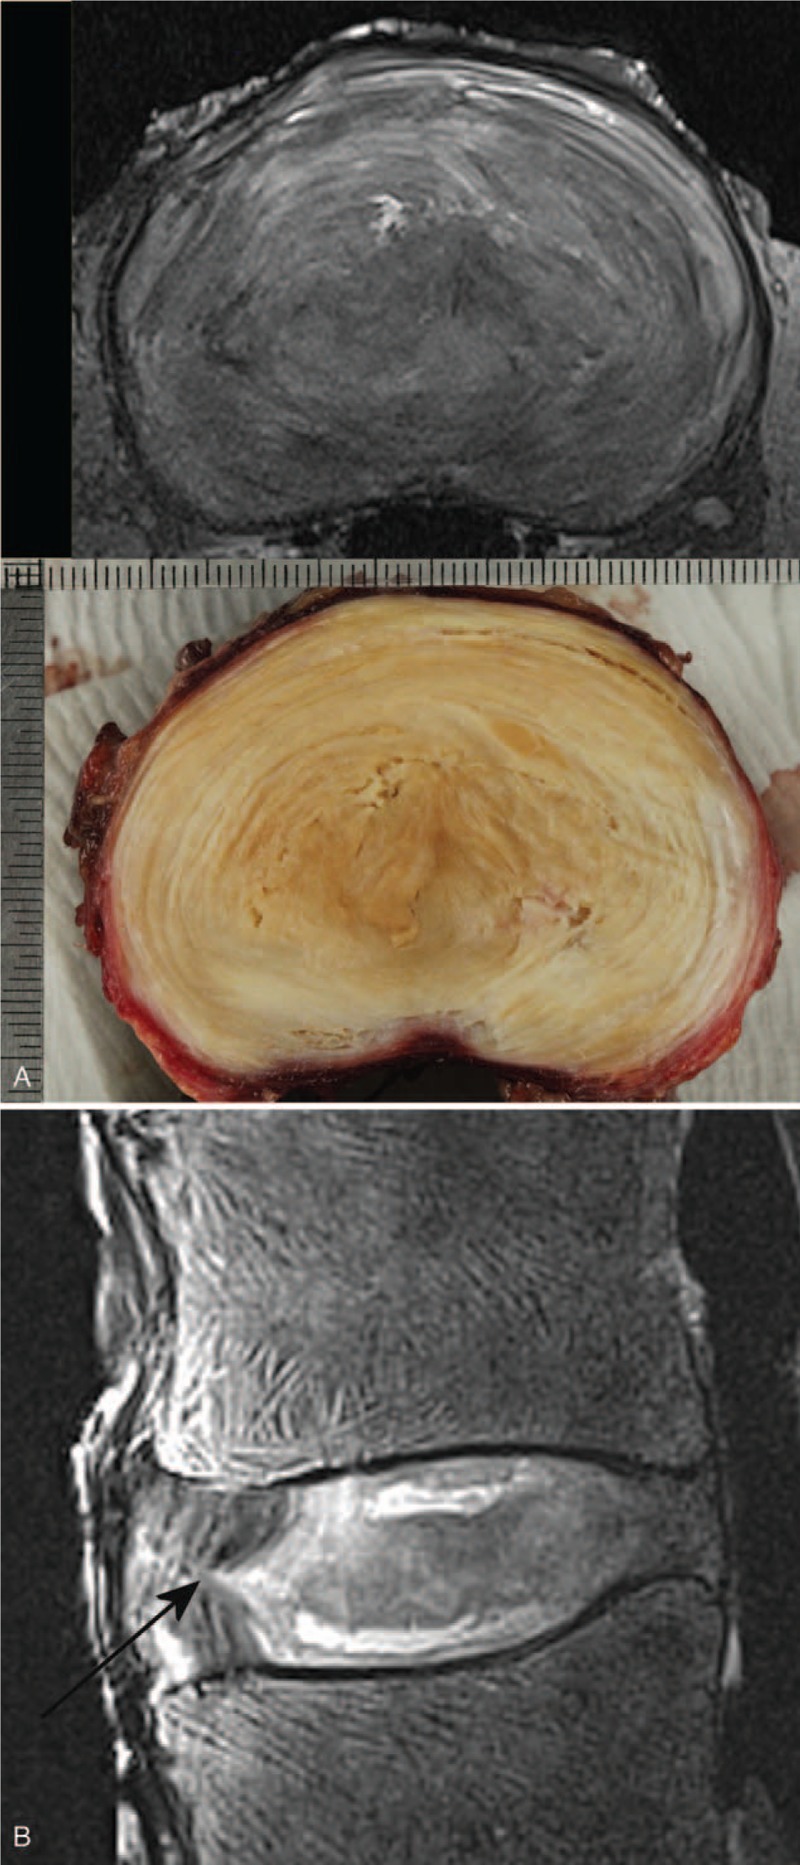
(A) Photograph of dissected section compared with 2D PD TSE transverse MR slice, and (B) 2D T2-weighted TSE fat saturated sagittal MR image of a L1L2 IVD. The anterior lamellae are drawn into the annular fissure (indicated by the arrow) in the sagittal plane (right anterio-lateral in the transverse plane). The fibrotic nucleus appears less intense, and fissures in the nucleus closer to the endplates appear bright. The vertebral-fluid rearrangement due to repeated freeze-thaw cycles are seen as a linear pattern of hyper-intense signals within the vertebral bodies on the sagittal image. IVD indicates intervertebral disc; TSE, Turbo-Spin-Echo.
